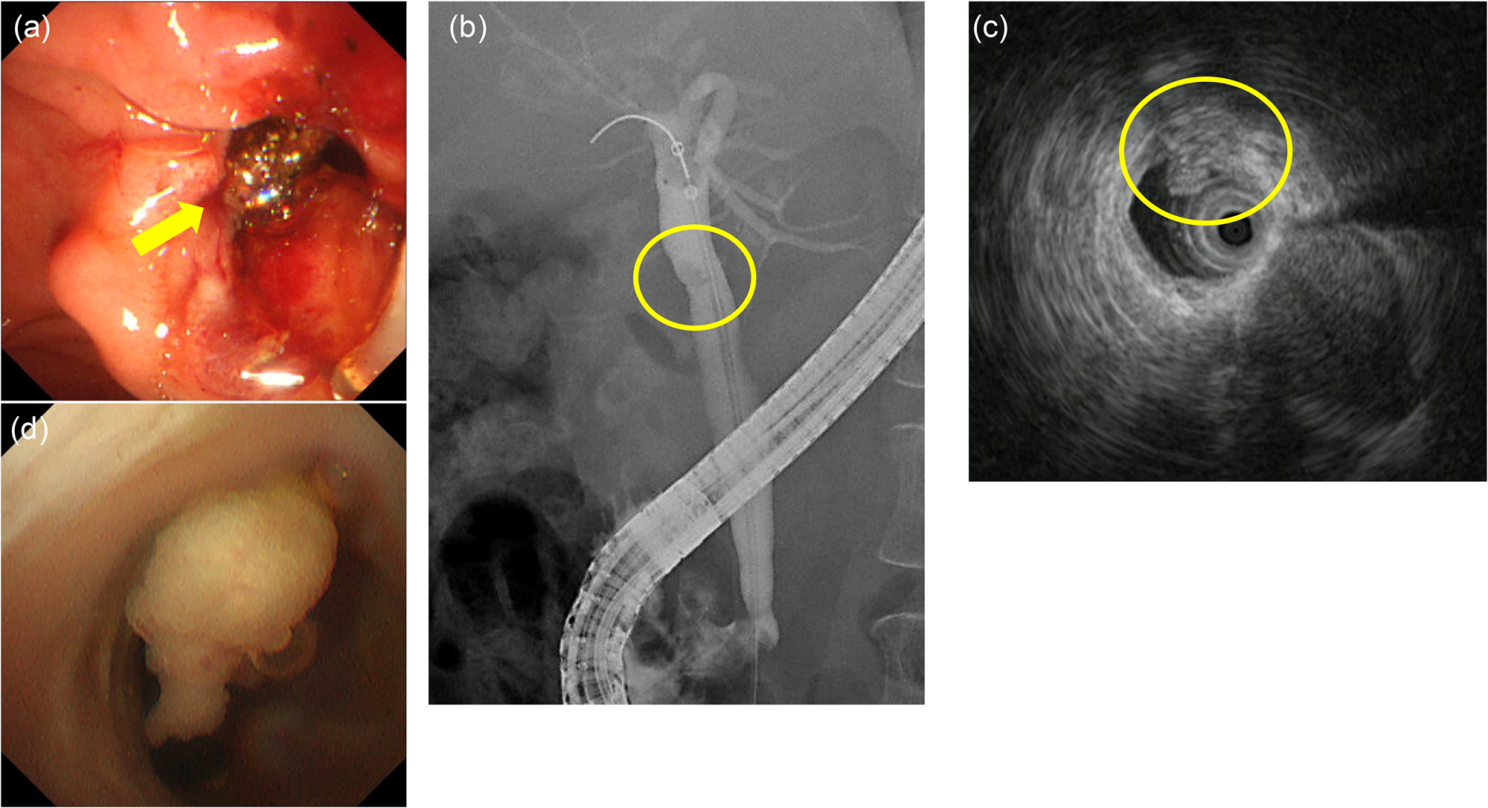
Endoscopic retrograde cholangiopancreatography. (a) To remove small common bile duct stones, an endoscopic sphincterotomy was performed (yellow arrow). (b) The cholangiography showed an immobile translucent image in the hilar bile duct (yellow circle). (c) The findings of intraductal ultrasonography imaging revealed that the tumor has invaded the bile duct epithelium, although the bile duct's exterior layer has been intact (yellow circle). (d) The peroral cholangioscopy examination showed a single white‐toned papillary tumor with an irregular surface at the same site
